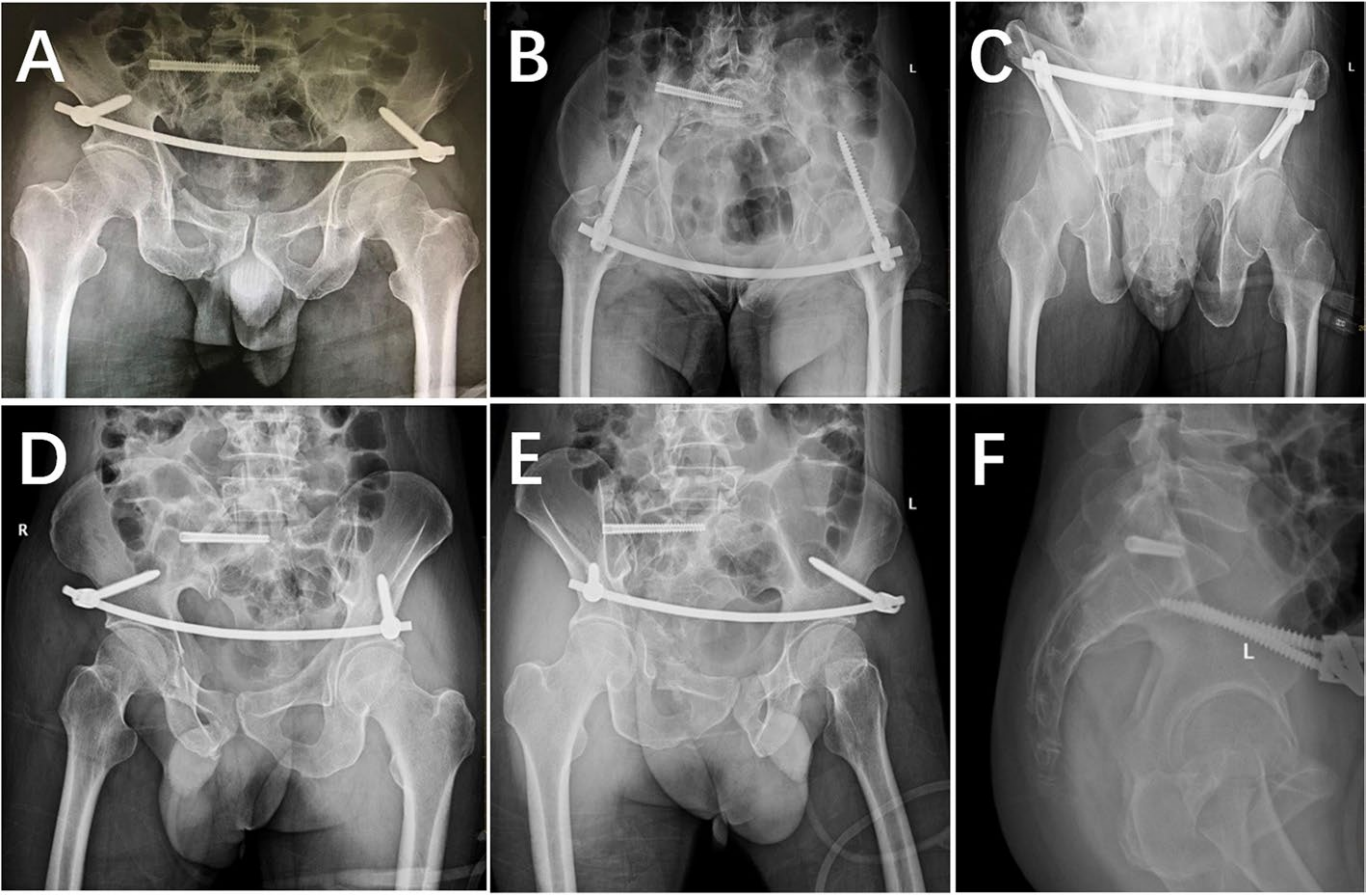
A–F: Postoperative day 3 radiograph showing well-reduced anterior and posterior rings with firm and well-positioned internal fixation
